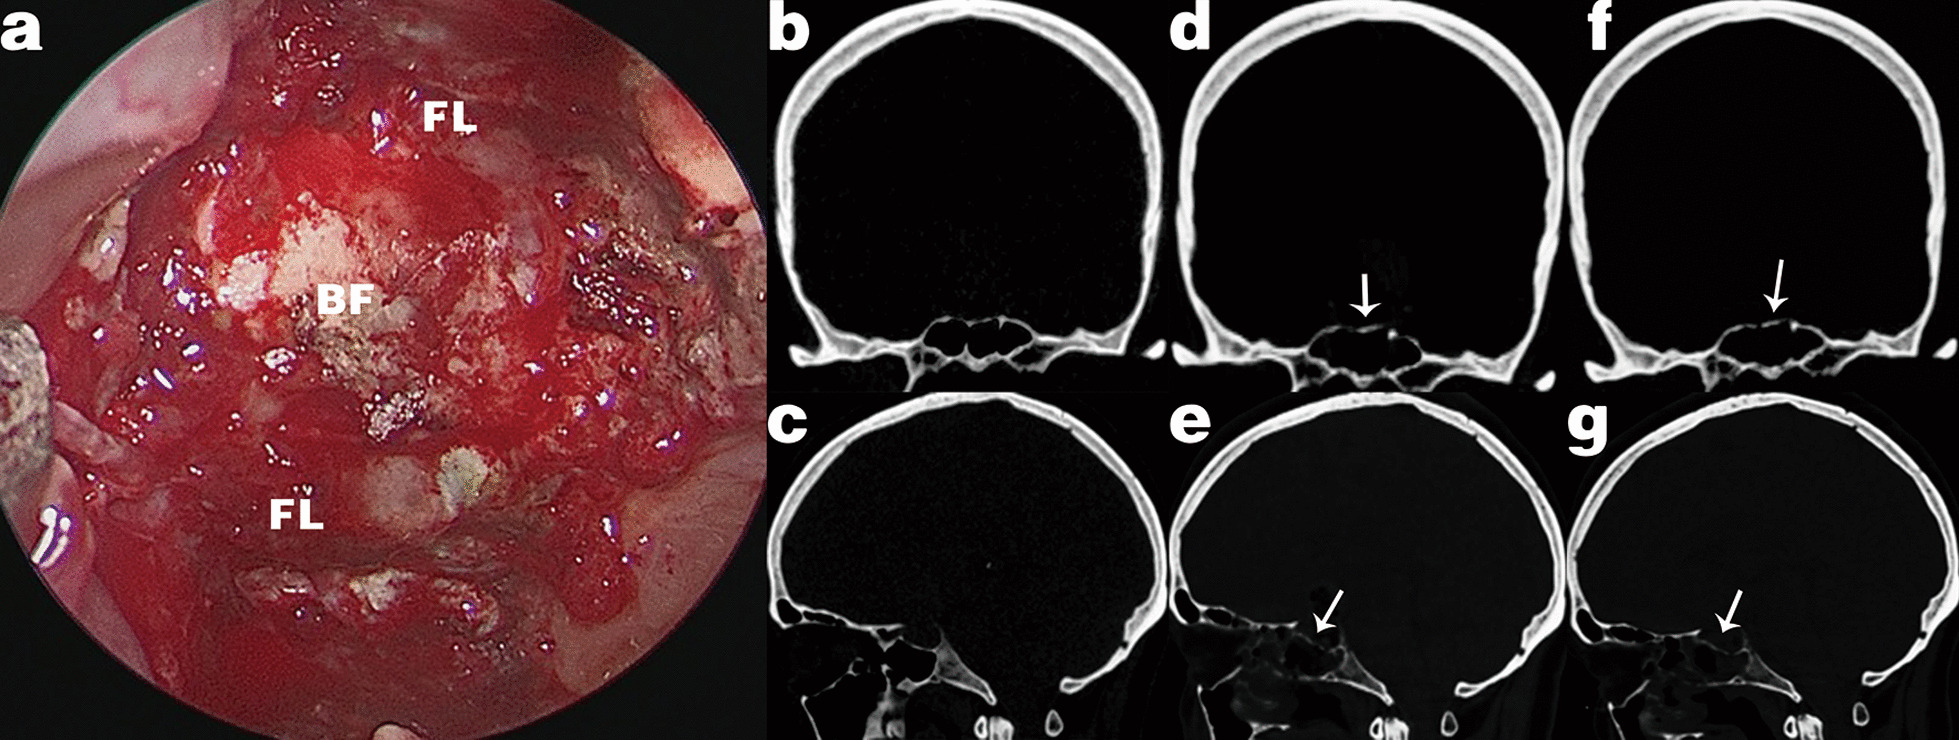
Representative postoperative images of CEFB outcomes. a During debridement under endoscopy 3 weeks after surgery, the bone flap and fascia are found to be in place and firmly attached to the defect. b Preoperative coronal and c sagittal CT images of the skull base bone structure. d Immediate postoperative coronal and e sagittal CT images of CEFB reconstruction. f Three months after surgery, coronal and g sagittal CT images demonstrate no dislocation or detachment of the bone flap. *BF* bone flap, *FL* fascia lata, Arrowhead = bone flap graft
